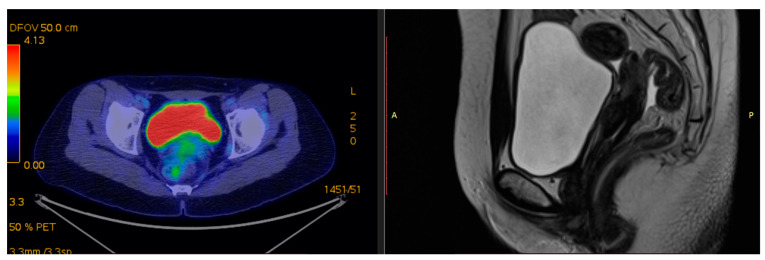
Post IGRT/CRT and IGABT treatment modalities in a FIGO IIIC1 case—left (axial view)—^18^FDG PET–CT low FDG uptake (SUV max 4) and right (sagittal view)—T2-weighted MR images—no mass in the cervix with low cervical signal intensity. Colposcopy with biopsy was negative for malignancy.
